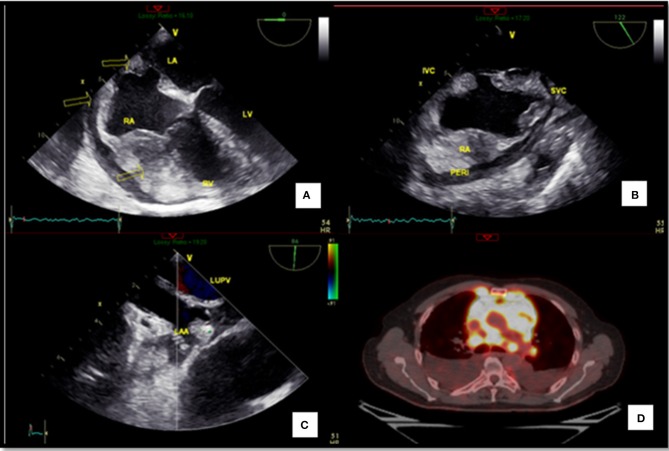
Extension of the lymphoma. (A) Transesophageal echocardiography revealing right ventricular infiltration. (B) Transesophageal echocardiography bicaval view showing an infiltration of the right atrium. (C) Transesophageal echocardiography (left atrial appendage with a dense round image that could be a thrombus). (D) PET-CT revealing the extension of the lymphoma. RA, Right atrium; RV, Right ventricle; LA, Left atrium; LUPV, Left upper pulmonary veins; LAA, Left atrial appendage; IVC, Inferior vena cava; SVC, Superior vena cava; PERI, Pericardium.
